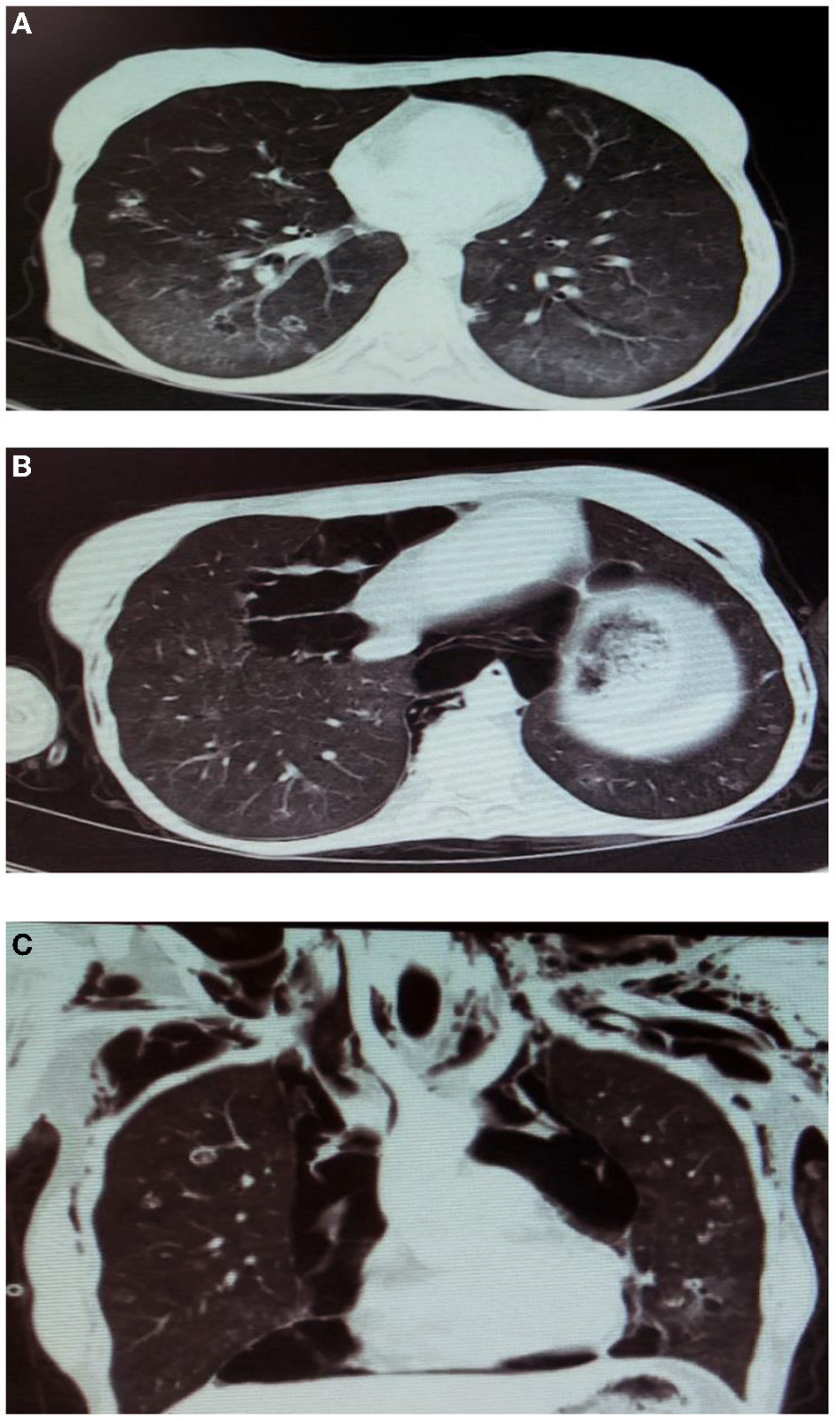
The spiral chest CT scan of the patient. (A) 1st day of hospitalization; bilateral ground-glass opacities and multiple nodules. (B,C) 12th day of hospitalization,pneumothorax, pneumomediastinum, extensive subcutaneous emphysema, and pneumopericardium.
